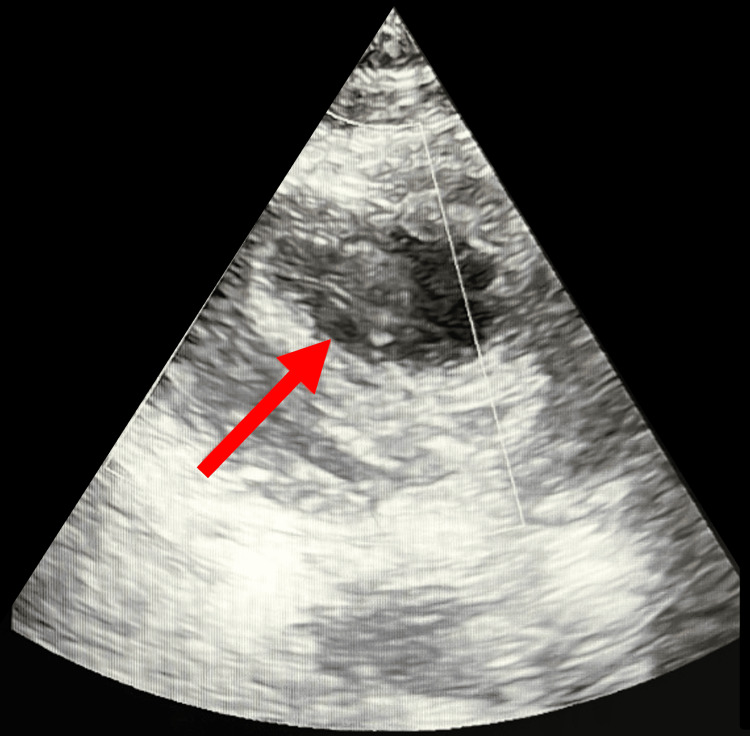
Transthoracic echocardiogram Two-dimensional transthoracic echocardiography demonstrating a homogeneous, echo-dense mass adherent to the anterolateral apical wall of the left ventricle (red arrow), consistent with a left ventricular thrombus in the setting of reduced left ventricular systolic function.
